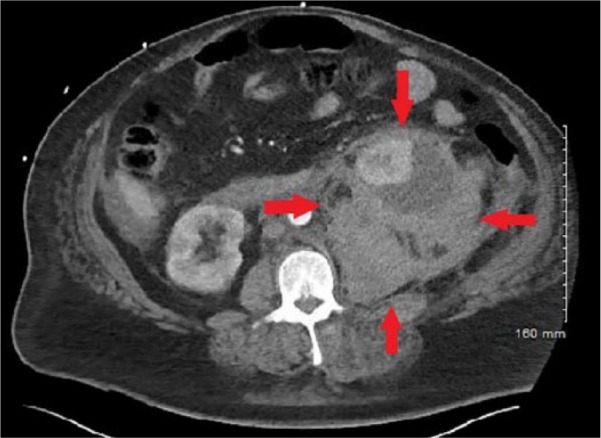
Computed tomography angiography scan of abdomen (axial view) showing retroperitoneal hematoma in the left suprarenal fossa causing mass effect on the left renal parenchyma.
